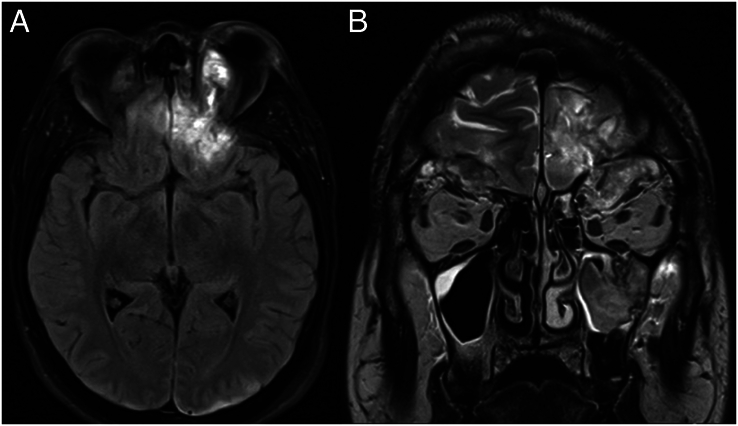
MRI scan on day 9 post-trauma demonstrating the traumatic intraorbital encephalocele in axial (A) and coronal (B) planes.
